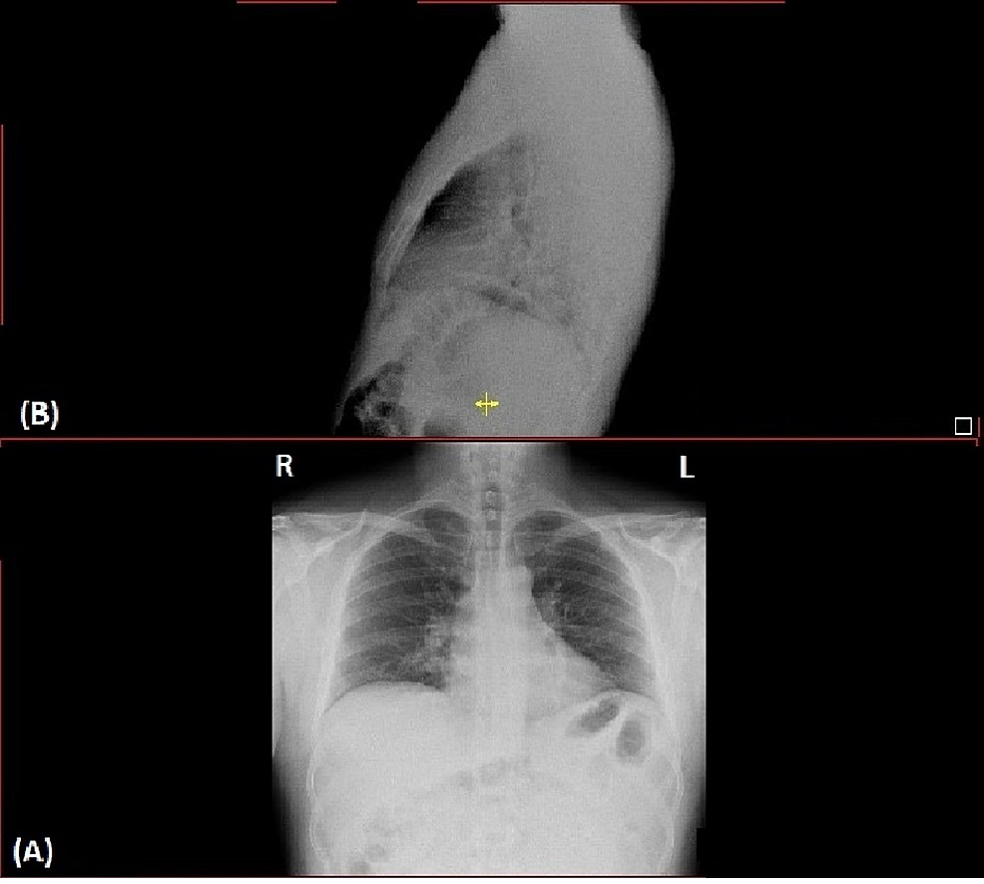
Chest X-ray: (A) lateral view and (B) anteroposterior view. R: Right, L: Left
